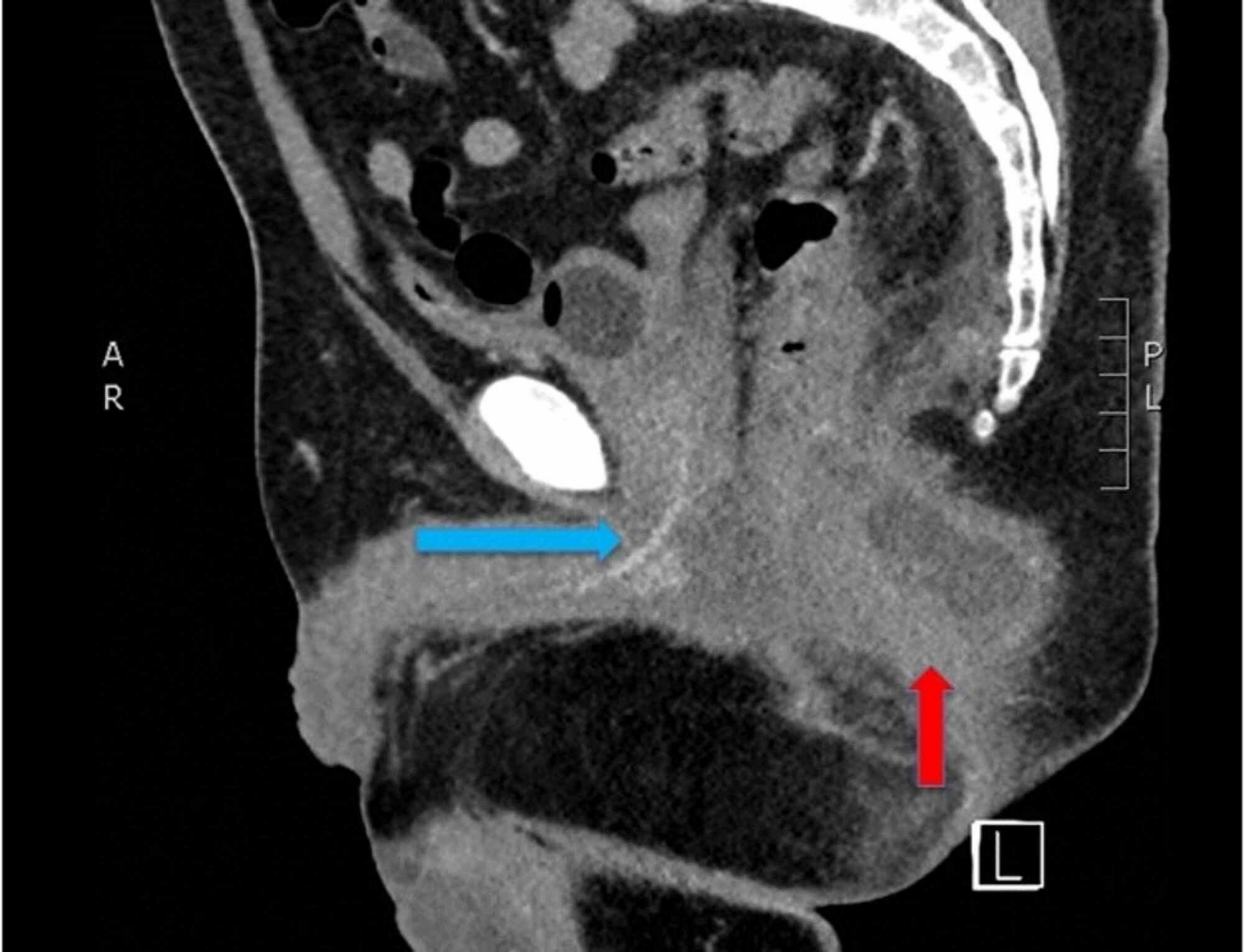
Sagittal Slice CT Pelvis with IV Contrast Ring enhancing lesion posterior to rectum (red arrow). And anterior is foley catheter (blue arrow).
